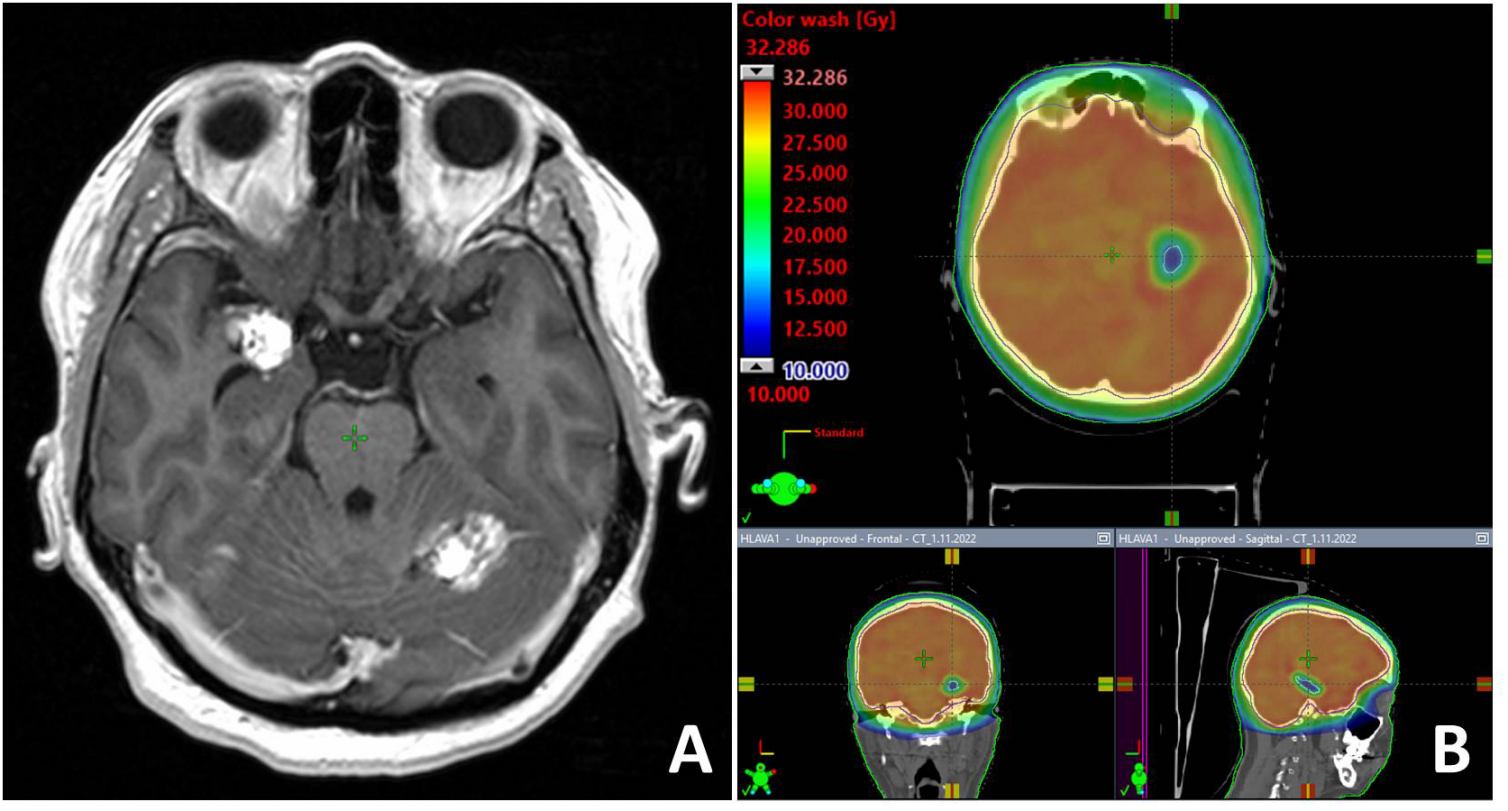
T1-weighted contrast enhancing MR examination with one metastasis located close to the right hippocampus (A). Unilateral hippocampal sparing radiotherapy technique (B) was employed in the palliative RT.
